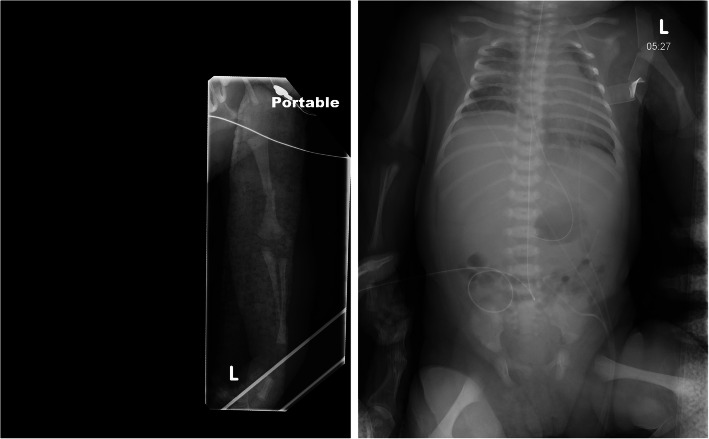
Left, Left midshaft humerus fracture on day of birth. Extension of the elbow and flexion and pronation of the wrist are evident and characteristic of Erb palsy. Right, Radiograph shows an elevated right hemidiaphragm, attributable to contralateral (right-sided) phrenic nerve injury
